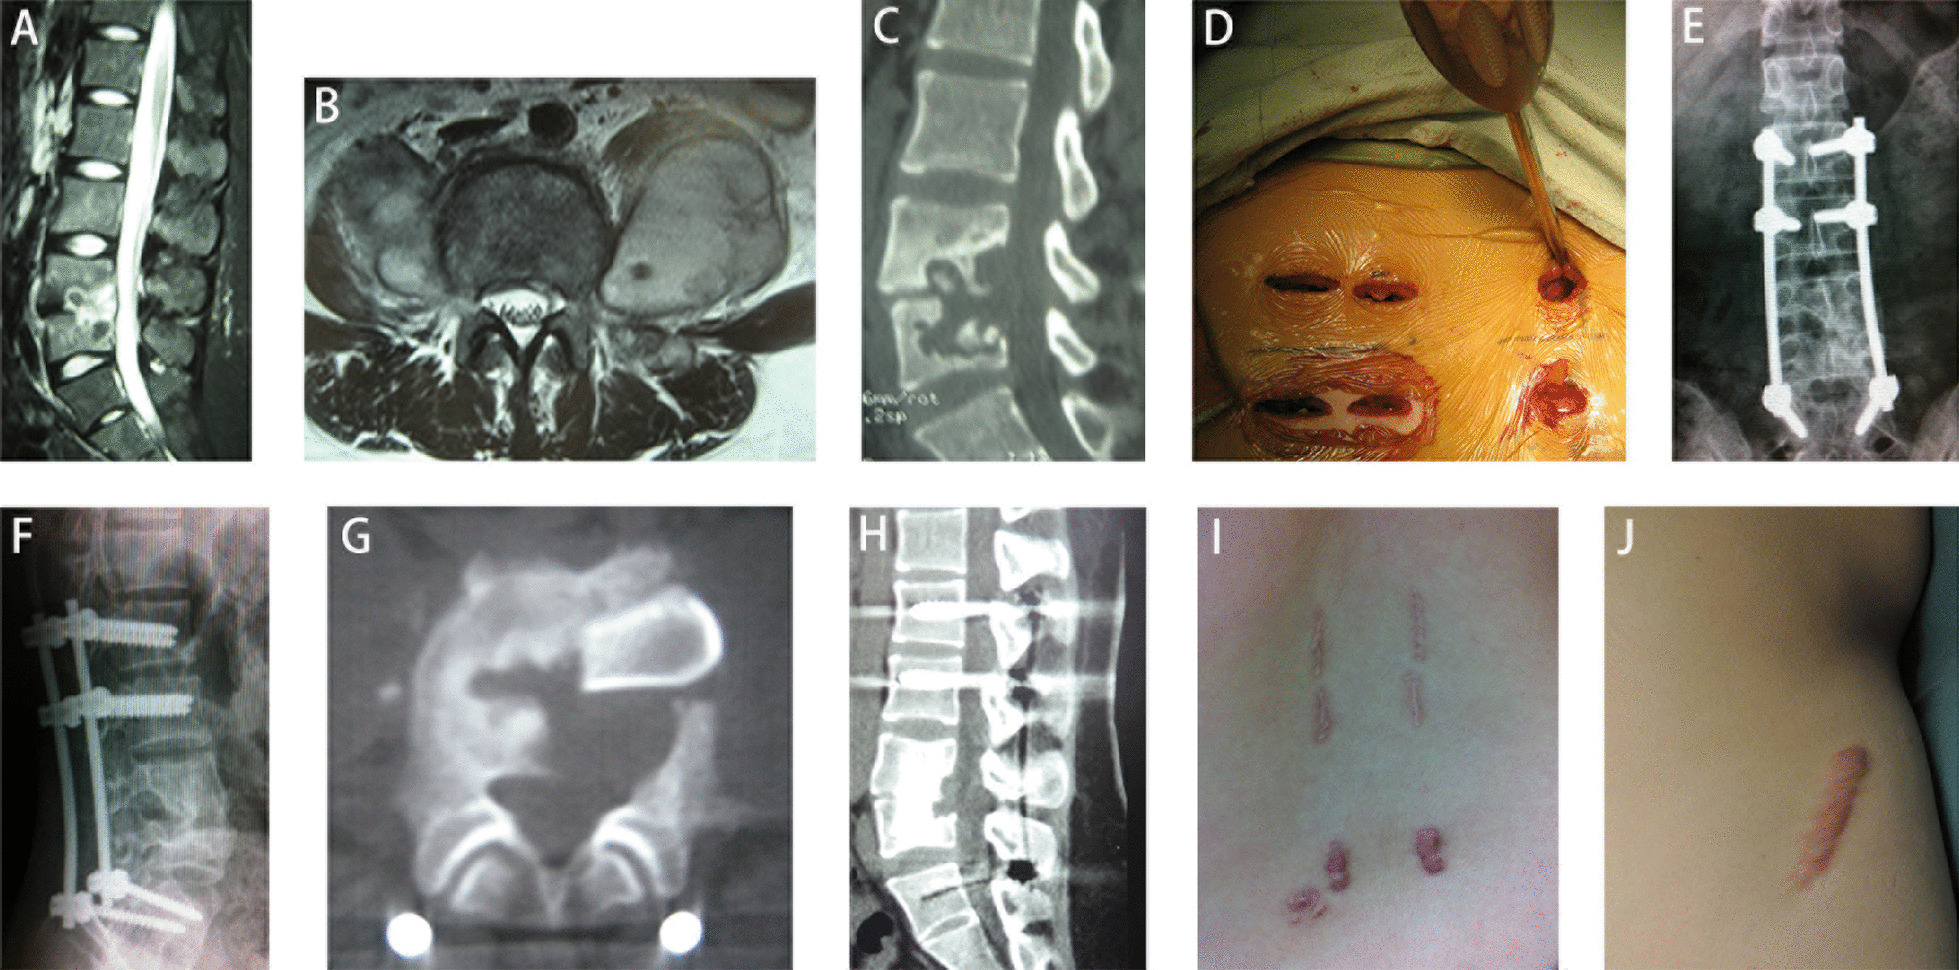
A 20-year male patient with L4–L5 tuberculosis accompany with psoas abscesses. Preoperative MRI (A, B) and CT (C) showed destruction of L4–L5 and psoas abscesses. Freehand MIPS (D). Postoperative x-ray (E, F) and CT (G) showed good location of the autogenous iliac bone and internal fixation. CT at 12-month after surgery (H) showed bone fusion achieved without internal fixation failure. Surgical incisions of freehand MIPS (I) and OLIF (J)
